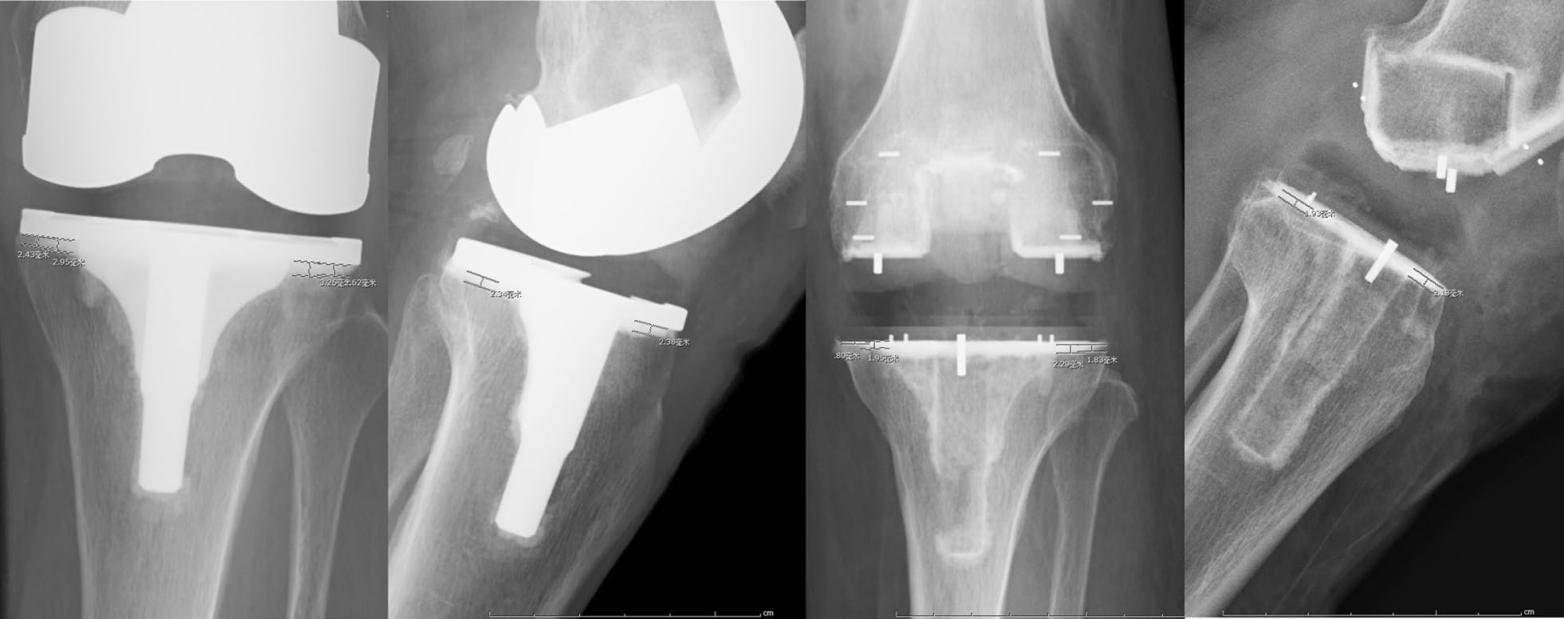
Anteroposterior and lateral radiographs with six zone cement mantle measurements
